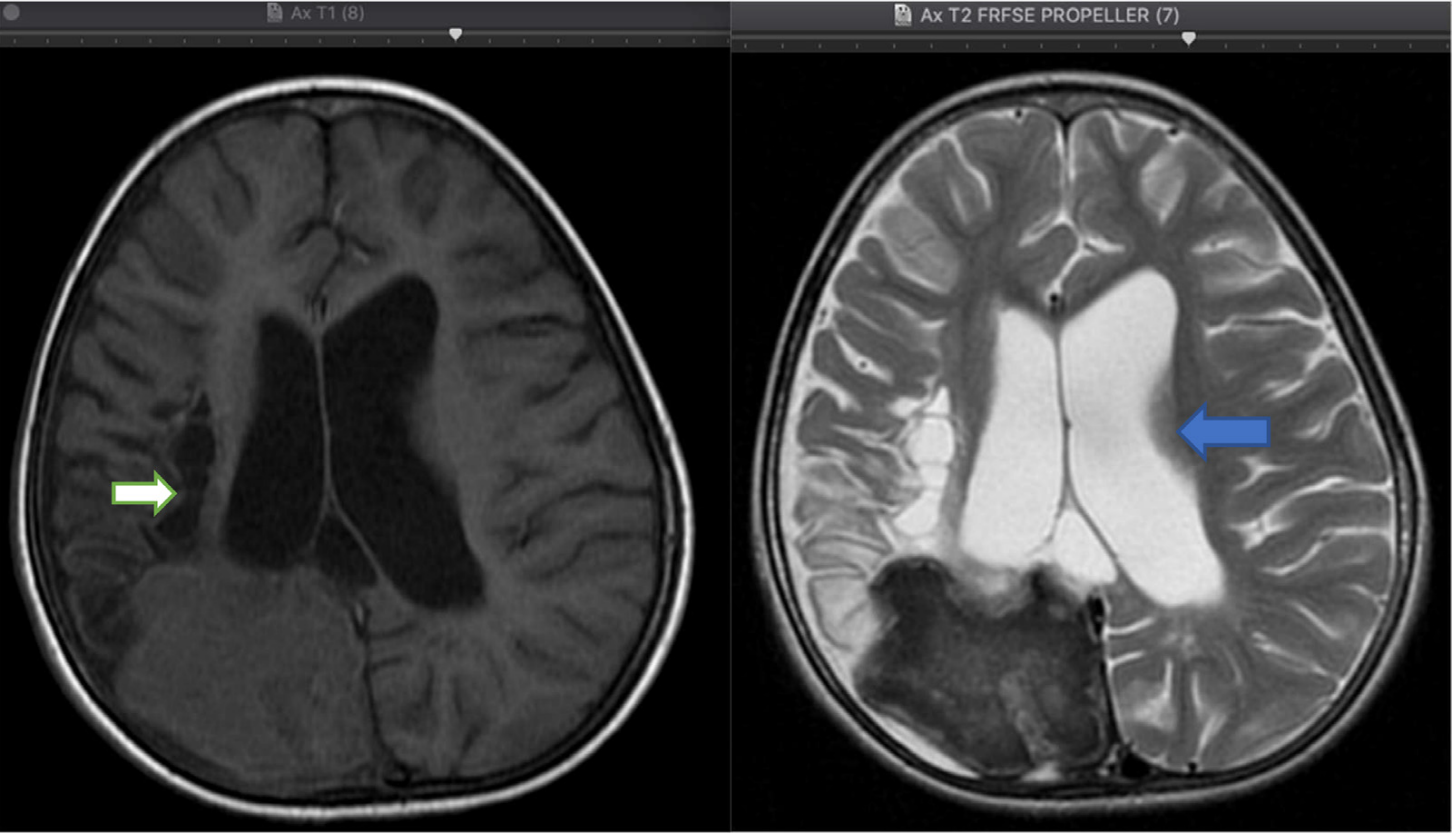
The axial section of the TI and T2 MRI shows multiple cystic lesions in the subcortical of the right parieto-occipital lobe representing *encephalomalacia cyst* (white arrow) with communicating hydrocephalus of the right and left lateral ventricles (blue arrow).
